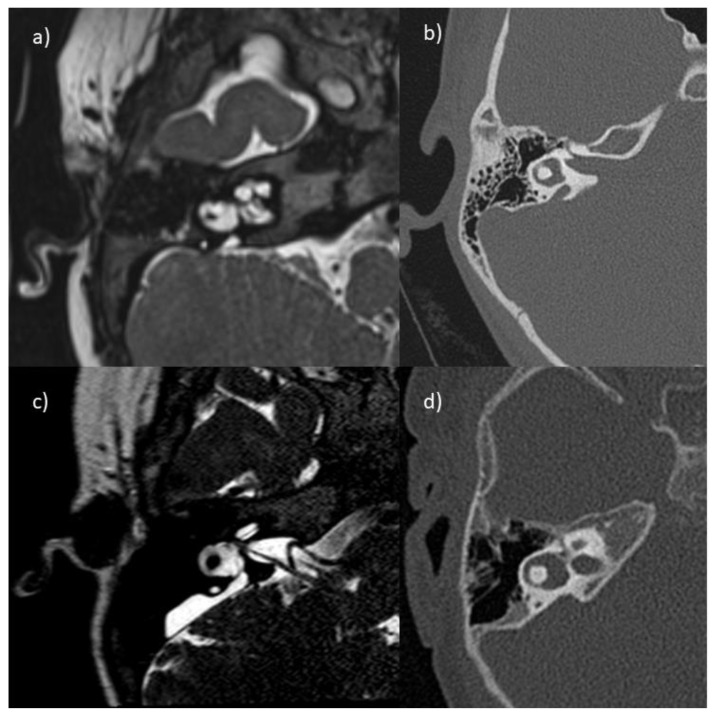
Images of right temporal bone in four children showing examples of dilated lateral semicircular canals at the ampulla portion (a–d) and a displacement of the bony center with a narrowing of the canal (a). Magnetic resonance imaging in (a,c), and computer tomography in (b,d).
